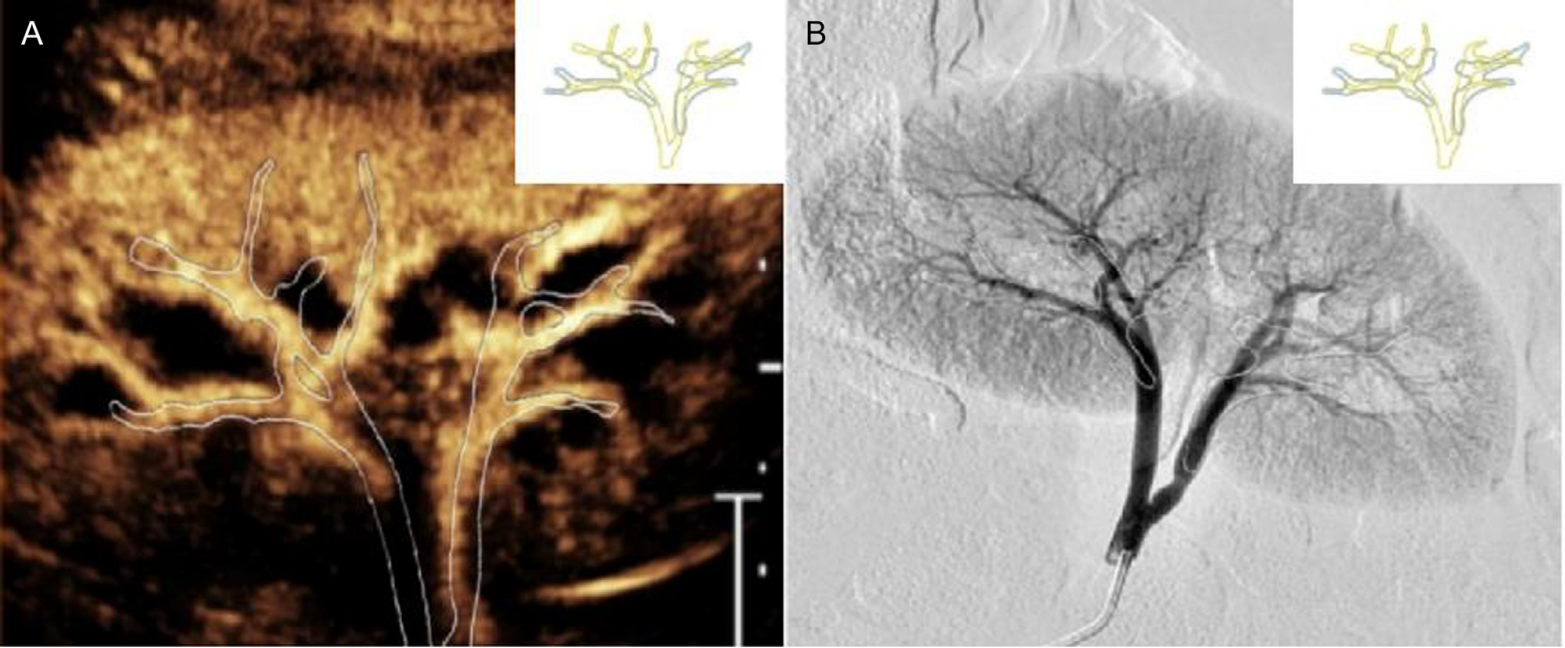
Visualization of renal cortical vasculature in a nondilated collecting system using (A) CEUS and (B) DSA. The vascular anatomy visualized by CEUS closely matched that observed in preoperative DSA, demonstrating consistent alignment of renal vascular structures. CEUS = contrast-enhanced ultrasound; DSA = digital subtraction angiography.
